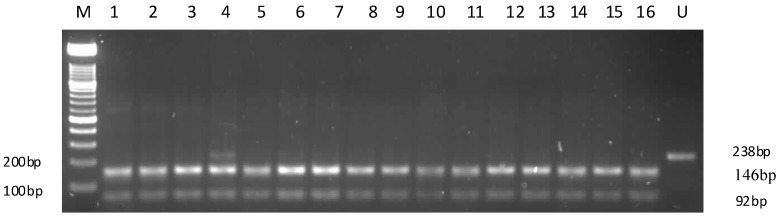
A representative 2% agarose gel of restriction digestion products with MspI for −482 of *APOC3* promoter region genotyping. The expected size of amplified product was 238 bp. Restriction patterns obtained with MspI were as follows: Lane M: 100 bp molecular marker; Lanes 1–3 and 5–16, wild type −482 CC genotype; Lane 4, CT, heterozygous genotype; and Lane U: undigested sample (no enzyme added). The presence of a restriction site is indicated by two or three bands.
